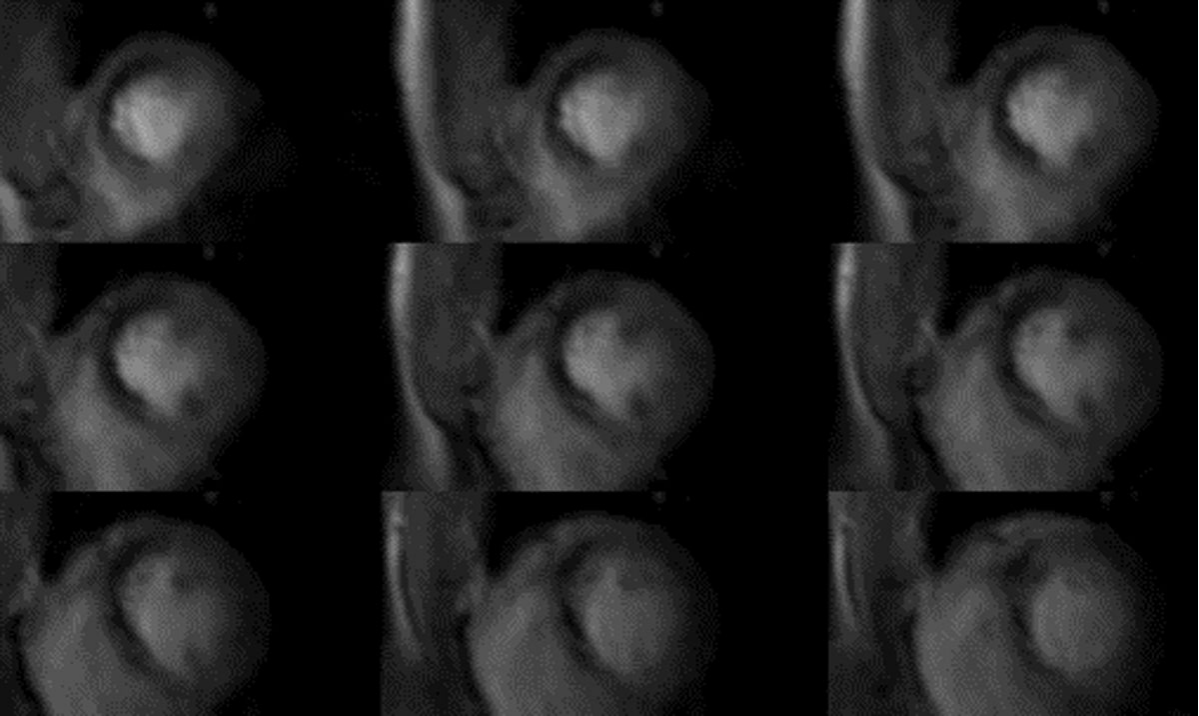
3-D CMR perfusion scans during adenosine stress showing a inducible perfusion deficit in anterior/anteroseptal segments extending from apical to basal slices. Invasive X-ray coronary angiogram demonstrated high-grade single-vessel disease with subtotal occlusion of the left anterior descending artery (LAD)
